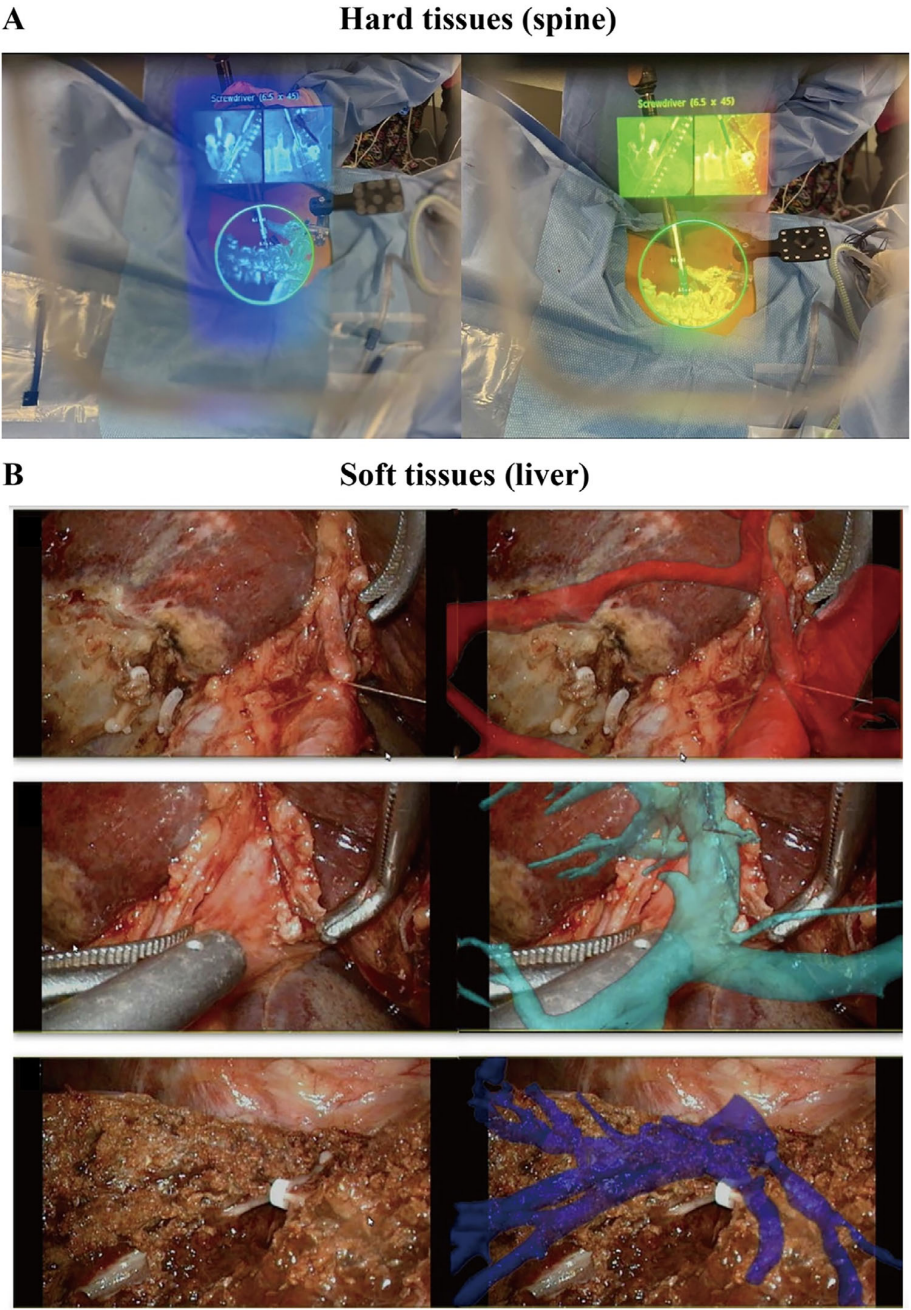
Augmented reality‐guided minimally invasive spine surgery and hepatectomy. A) Integration of 3D reconstruction of the spine with axial and sagittal CT images into the surgeon's field of view, resulting in a “see‐through” effect during spine surgery. B) Utilization of 3D reconstruction and navigation of crucial anatomical structures such as the hepatic artery (Red), portal vein (Green), and hepatic vein (Blue) during hepatectomy. Reproduced with permission. Copyright 2023, Elsevier and 2022, American College of Surgeons.^[^
